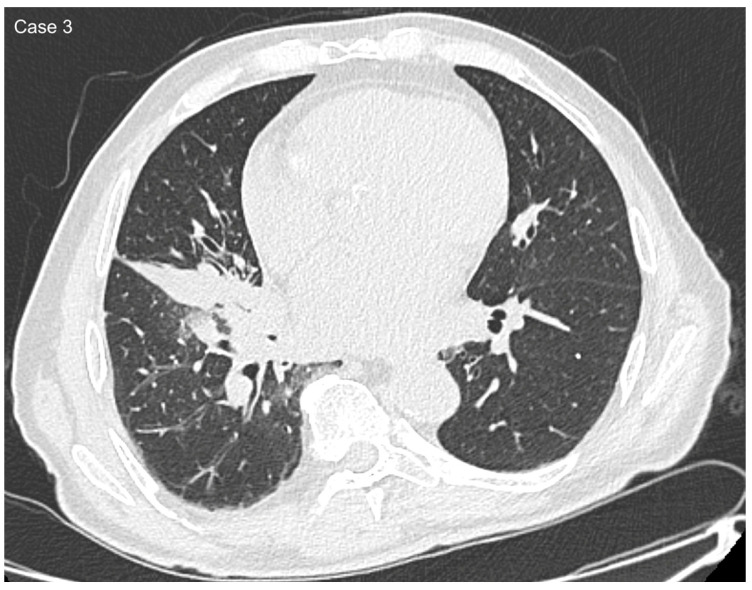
Axial CT image shows discrete ground-glass changes surrounding the lesion, as well as subsegmental collapse due to infiltration of the lateral bronchus of the middle lobe.
